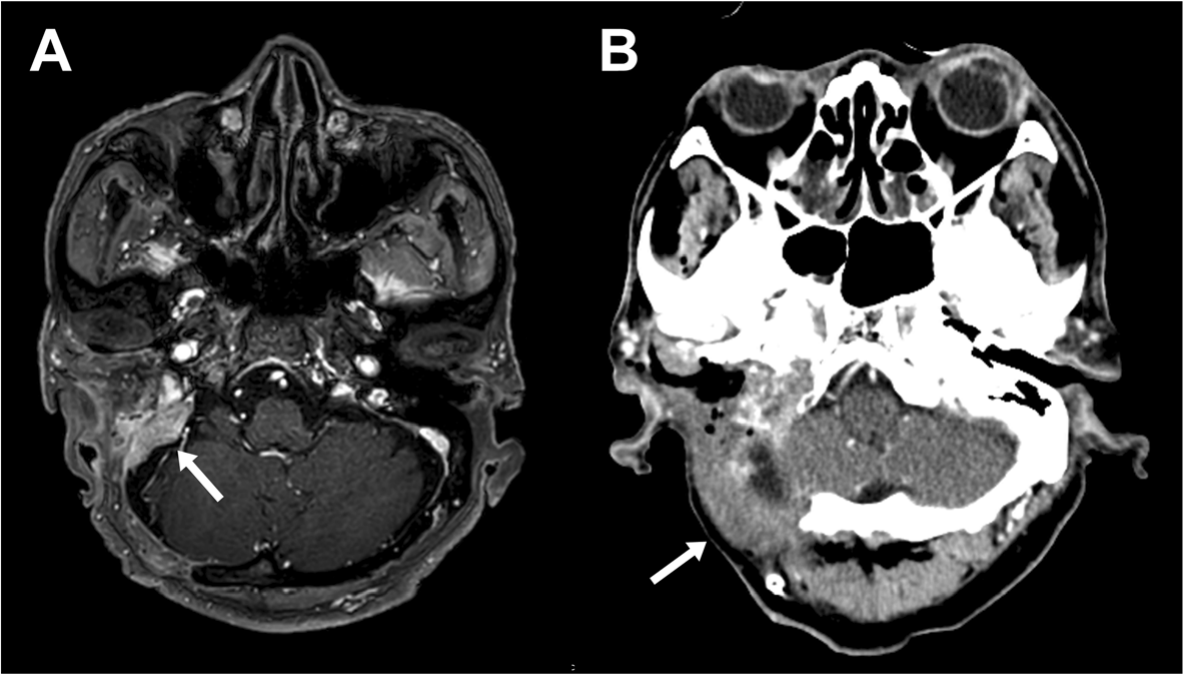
Postoperative magnetic resonance imaging (a) shows a small residual tumor around the jugular foramen, and computed tomography scan (b) demonstrates the sternocleidomastoid muscle which fills the tumor removal space
